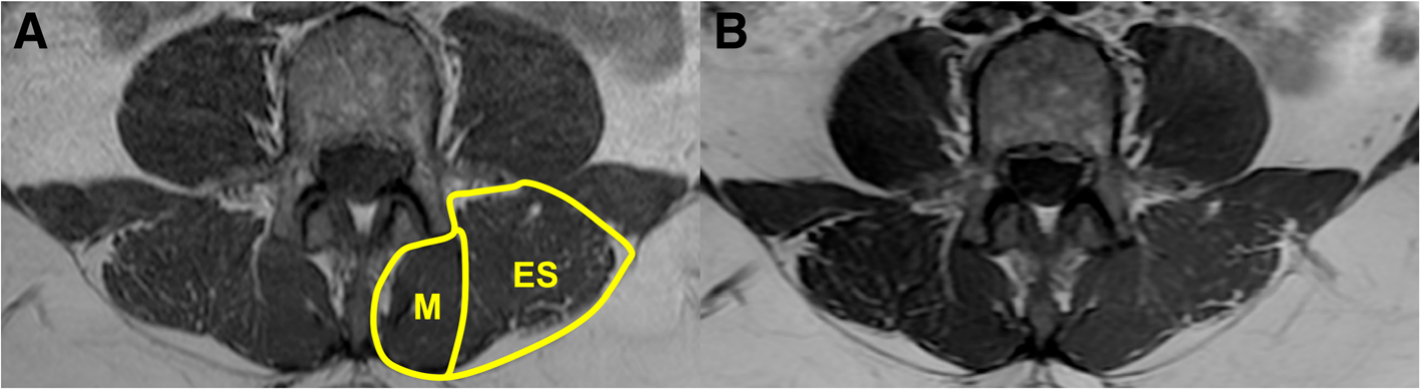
Example pre- (a) and post-rehabilitation (b) MR images of the lumbar musculature. The erector spinae (ES) and multifidus (M) muscles have been manually defined for the left side of the patient in A
